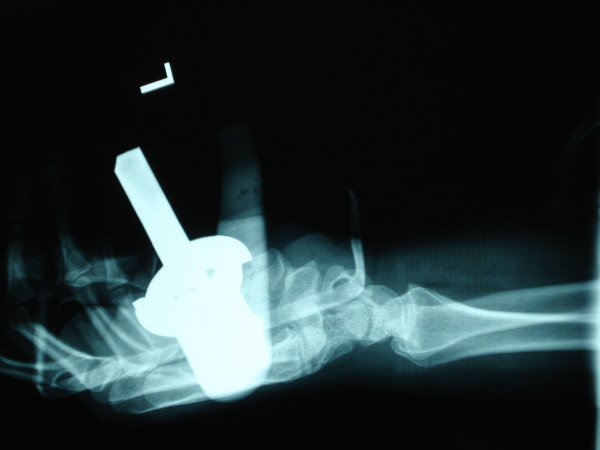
Lateral radiograph of hand.
